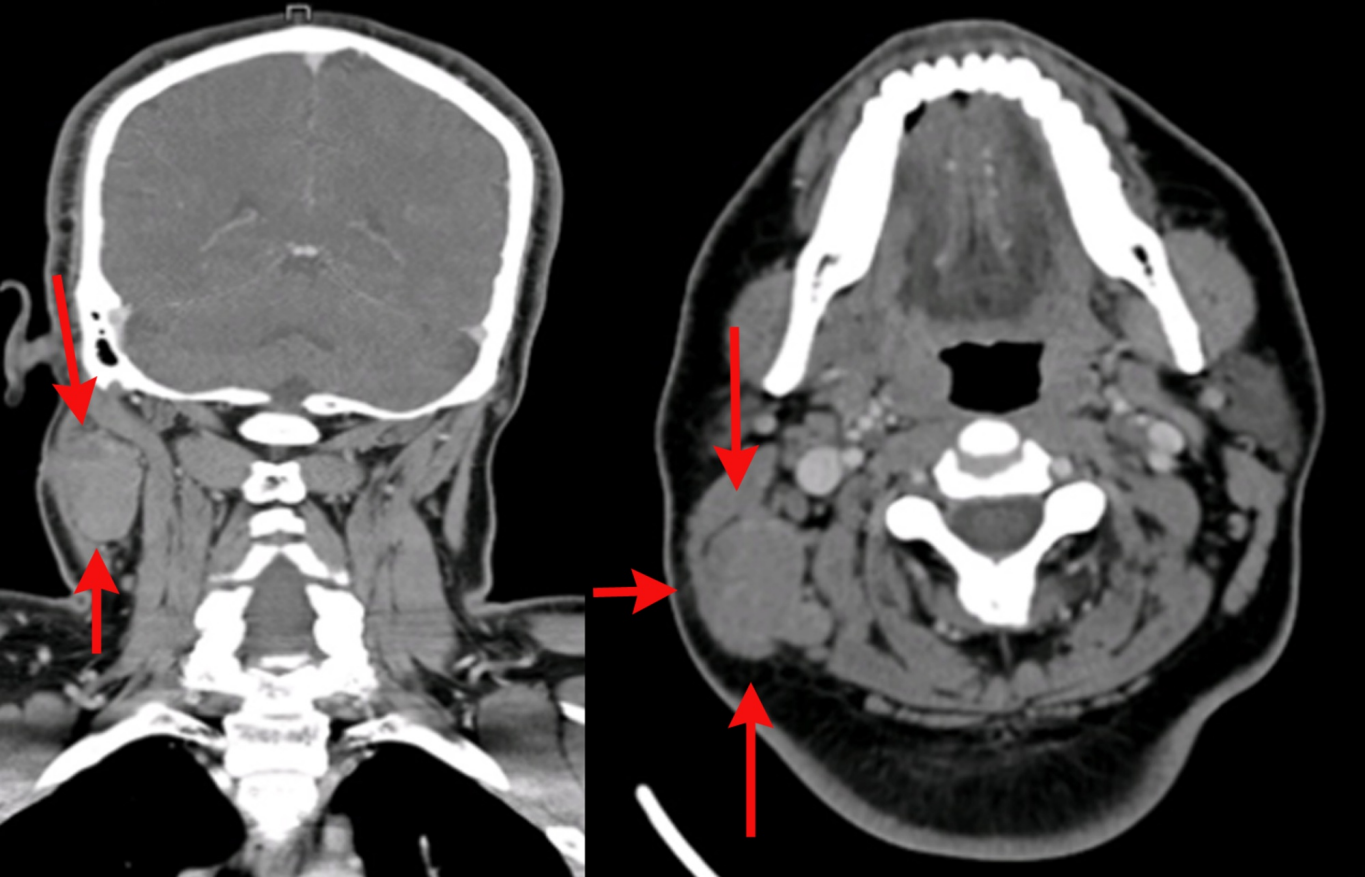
Computed tomography scan showing enlarged lymph node on right side of the neck (red arrows)
